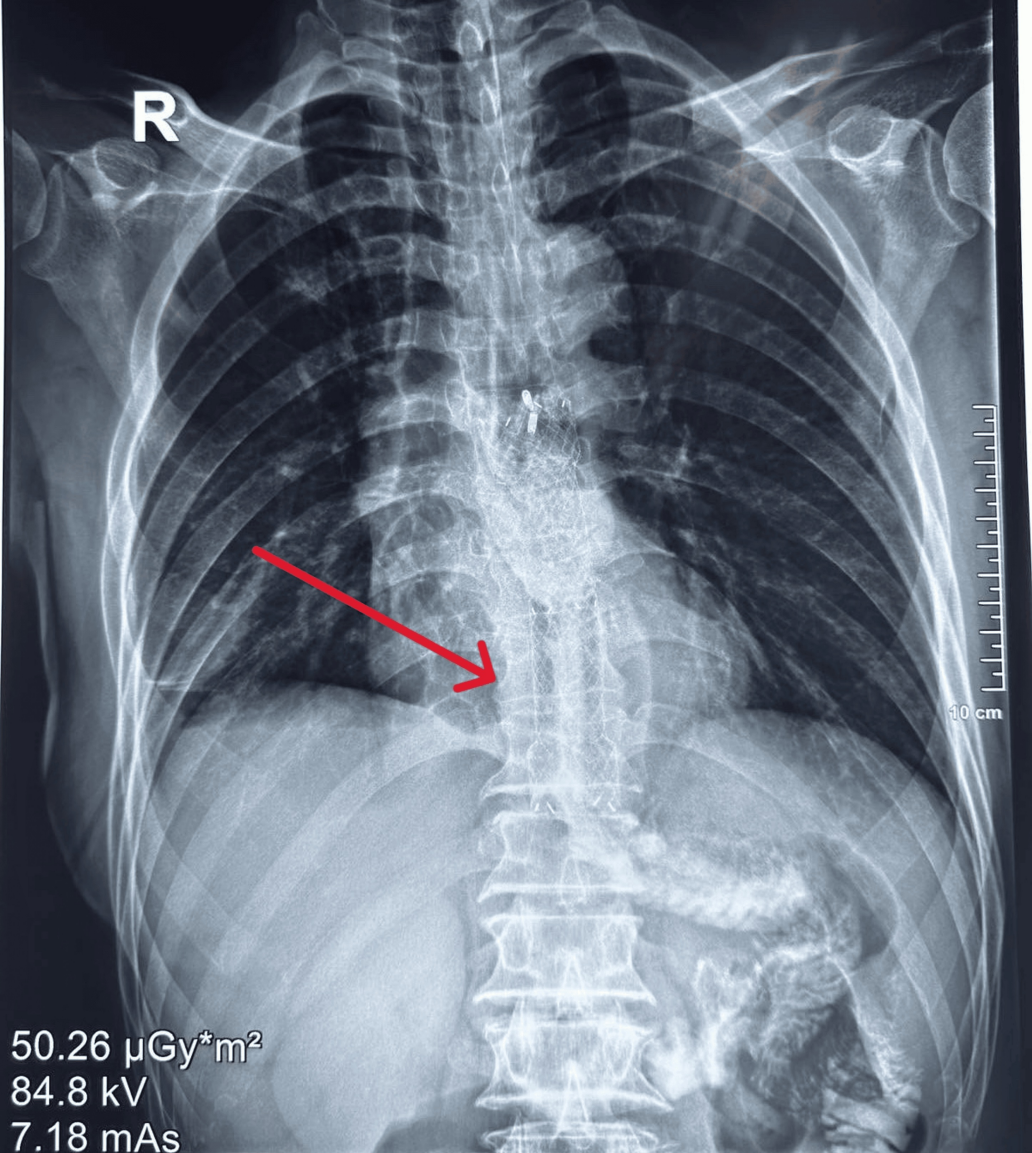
Chest X-ray after administration of water-soluble contrast medium showing no further leakage.
